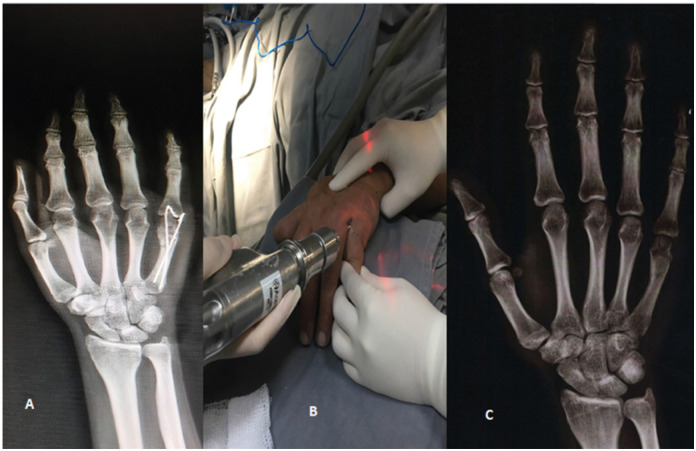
A) Anteroposterior radiograph in the immediate postoperative period of fixation of the 5° metacarpal diaphyseal fracture with FK. B) Retrograde intramedullary fixation technique with FK. C) Anteroposterior radiograph - Consolidation of the 5° metacarpal diaphyseal fracture.
